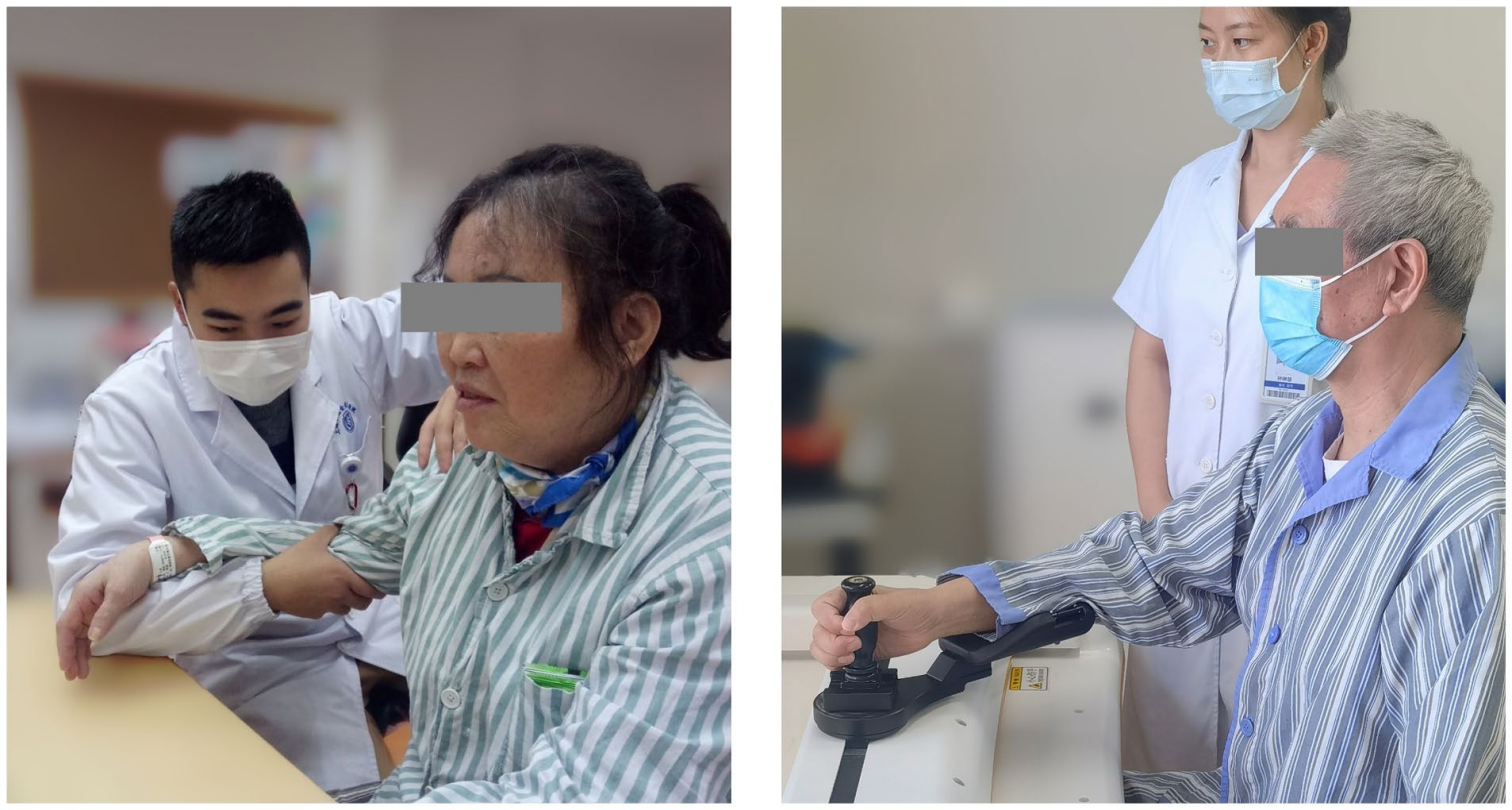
The actual scene of intervention during CT (left) and RT (right).
